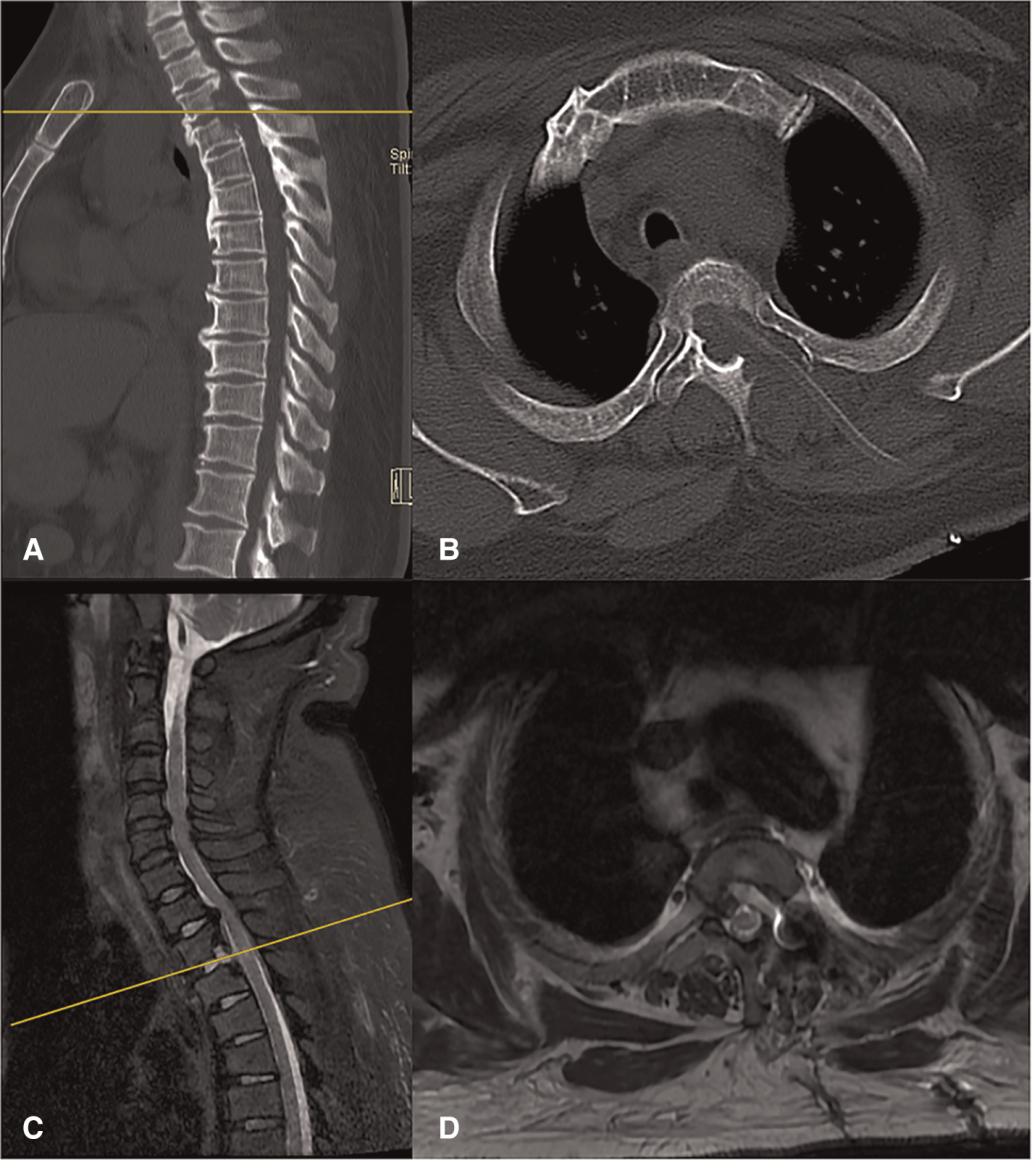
Postoperative CT (A,B) and MRI (C,D) showed the most TOPLL was excised and the thoracic spinal cord was completely decompressed.
